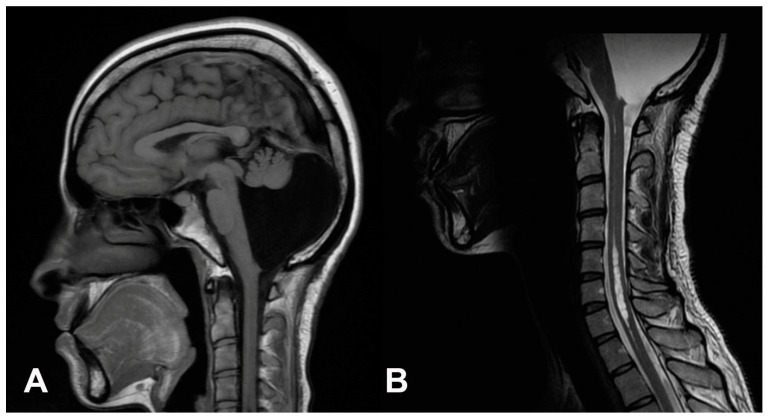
Preoperative MRI. (A): Brain MRI showing normal ventricle size without hydrocephalus. The cyst occupies nearly the entire posterior fossa, alongside hypoplastic cerebellar vermis; (B): cervical spine MRI showing syrinx extending from C4 to T1 vertebral levels.
